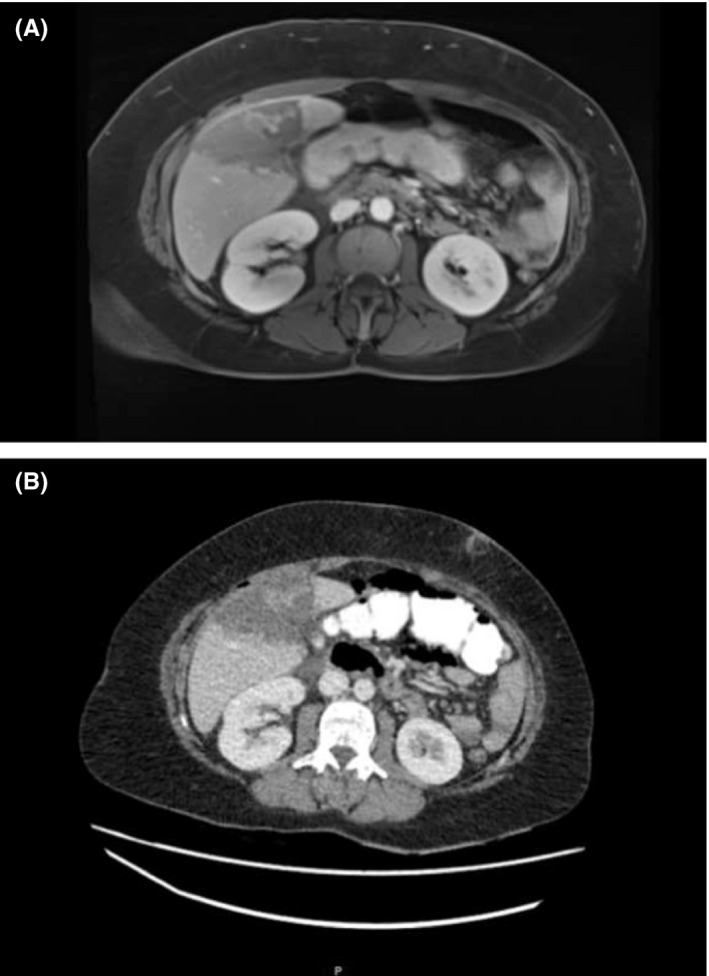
A, MRI venous phase—–a well‐defined necrotic area between segments 4 and 5 as shown in CT scan; margins are more clear delineating an hypointense zone where normal hepatic vein branches are seen. B, CT venous phase—a well‐defined necrotic area between segments 4 and 5 visible as an hypodense zone with clear margins
